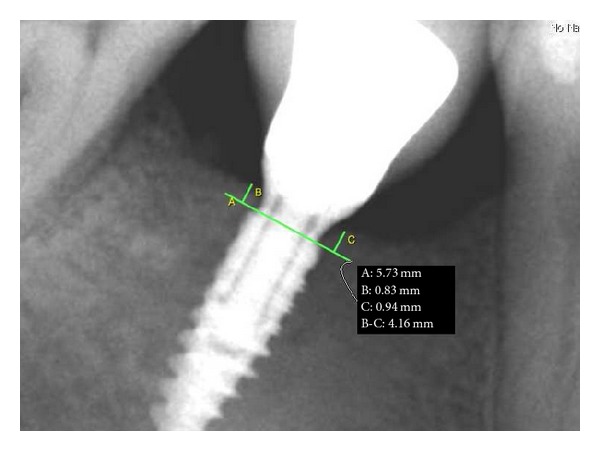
One-year radiographic follow-up.
